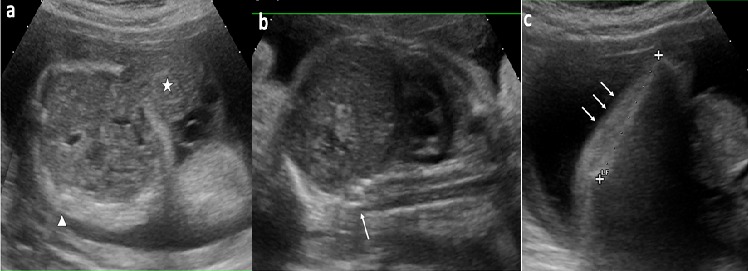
Obstetric ultrasound; a): axial section of the fetal abdomen, omphalocele with gray content (star), surrounded by a thin wall where passes the umbilical vein; The collar measures : 1.5 cm (dotted); b): in strict sagittal section of the fetus, abrupt interruption of the dorsal spine with no visualization of the lumbosacral and coccygeal segments (arrow); c): centered on the right lower limb, anomaly of the echogenicity of the lower limb with dedifferentiation of the musculoskeletal plans (arrows)
